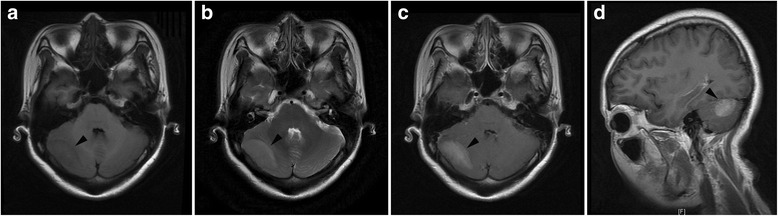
Axial T1 and T2-weighted images showed mass lesions in right cerebellum (*black arrowhead*), which displayed enhancement in enhanced axial T1 and sigttal T1-weighted images. a Axial T1WI. b Axial T2WI. c Enhanced axial T1WI. d Enhanced sigttal T1WI
